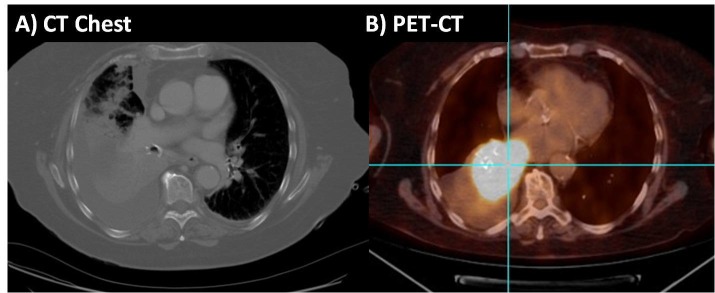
A) Chest computed tomography (CT) scan demonstrating right lower lobe consolidation with moderate to large sized right pleural effusion and B) positron emission tomography-computed tomography (PET-CT) scan demonstrating increased fluorodeoxyglucose (FDG) uptake in the heterogeneous right lower lobe pulmonary mass involving bronchus intermedius and right lower lobe bronchus
